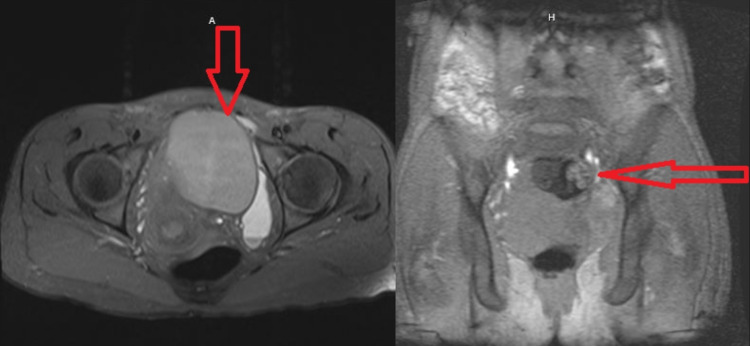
MRI chest/abdomen/pelvis MRI findings suggestive of an endometrioma measuring 7.2 × 7.1 × 4.2 cm in the mid-upper pelvis abutting and pushing the uterus to the right (red arrow, left). Moderate amount of hemorrhagic fluid in the pelvis and bilateral paracolic gutters with loculated left pelvic fluid measuring 9.3 × 3.3 cm (red arrow, right). A: anterior, H: hilum and mediastinum.
